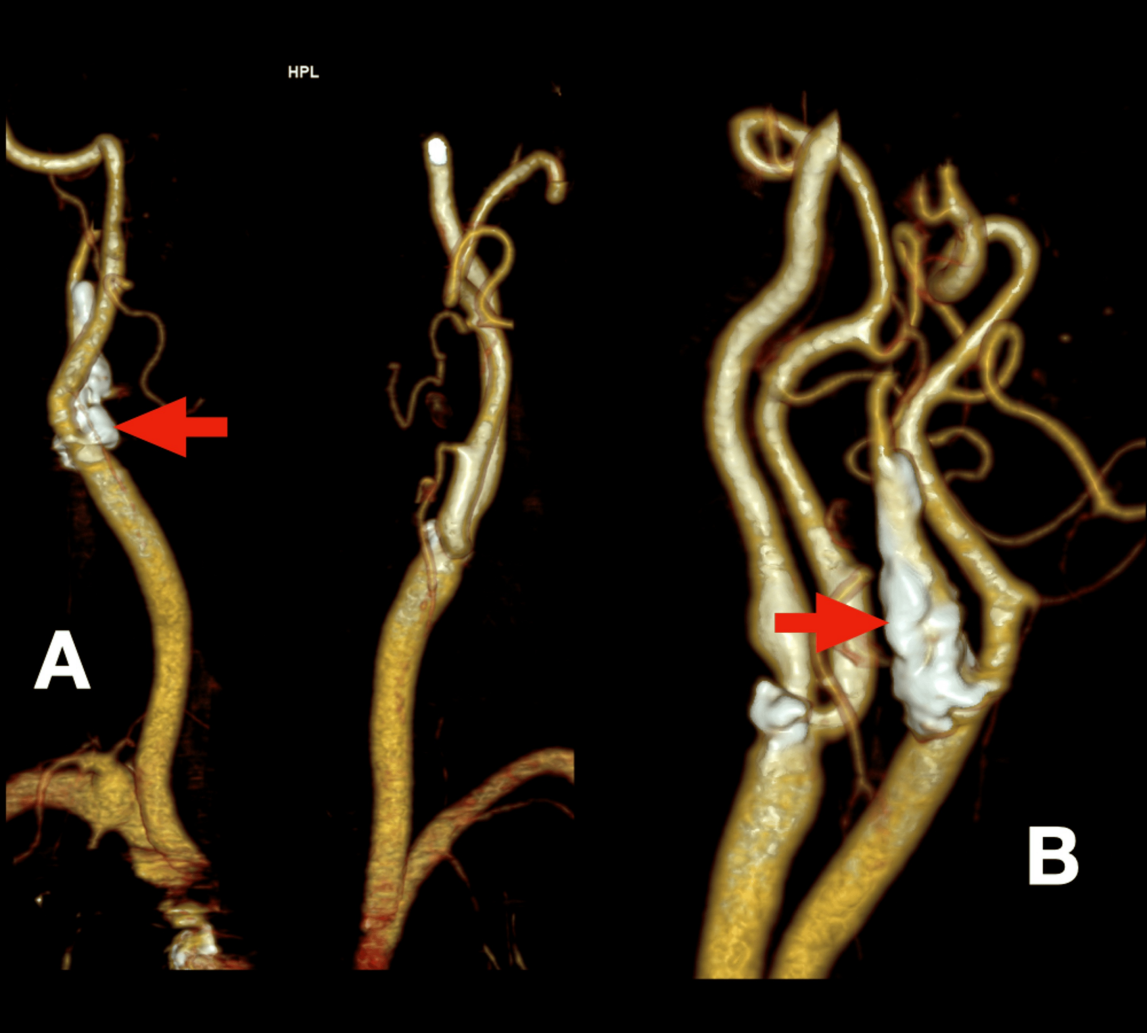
Reconstruction of cervicoencephalic CT angiography Red arrows in panels A and B show, from different perspectives, calcified atherosclerotic plaques involving the bifurcation of the right common carotid artery and the proximal right internal carotid artery
